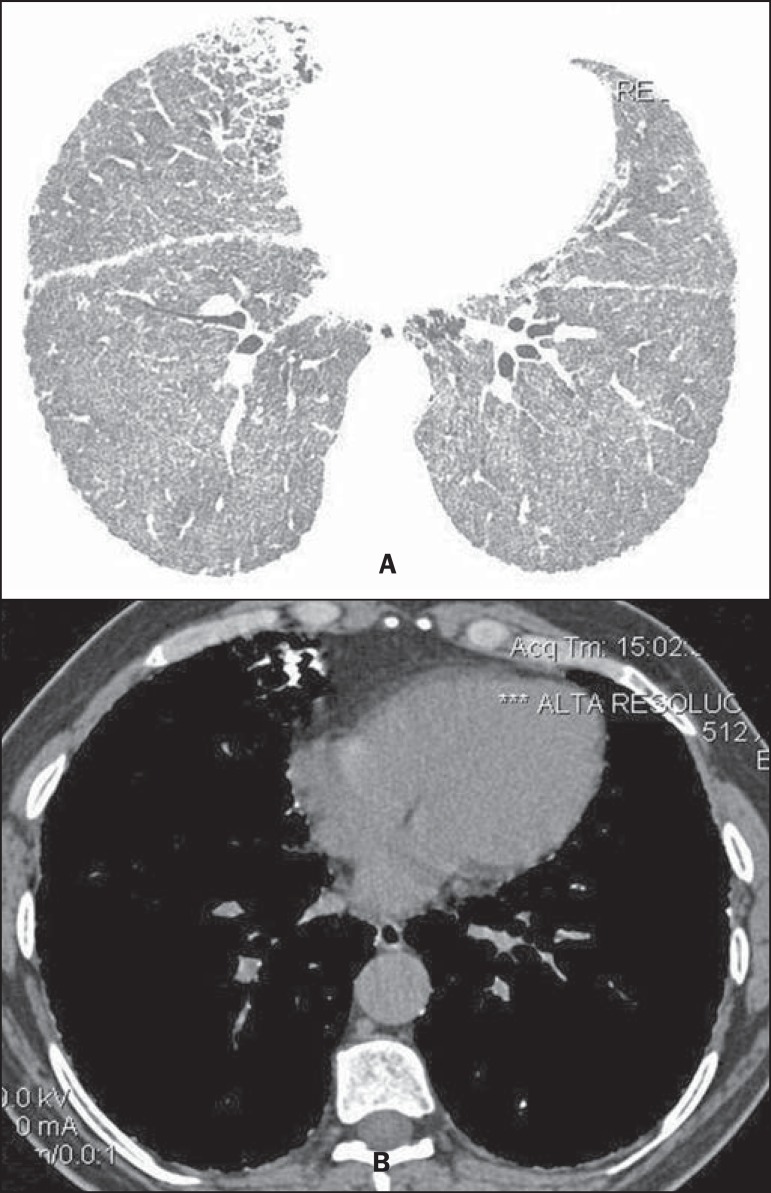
HRCT with lung windows setting shows (A) diffusely distributed ground
